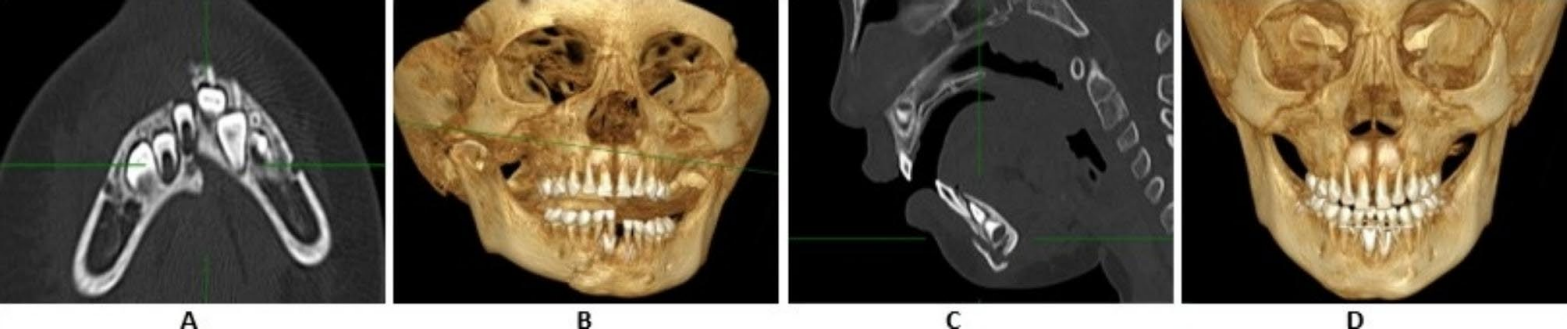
3-D CBCT showing A-C. Patient having mental fracture with obvious dislocation D. patient having mental fracture pre-operative after 6 months
